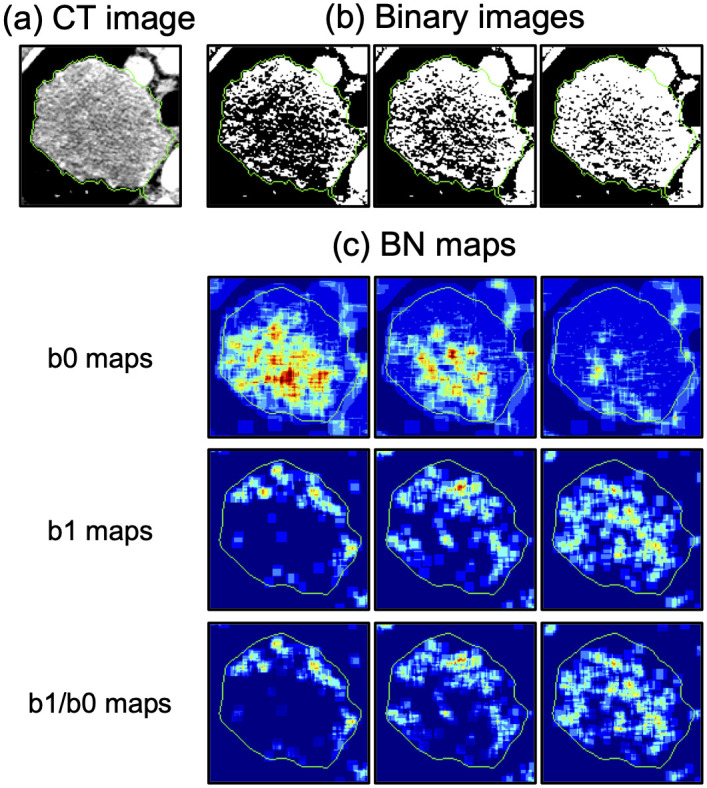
Representative images of (a) computed tomography (CT) images, (b) binary images, and (c) Betti number (BN) maps.
